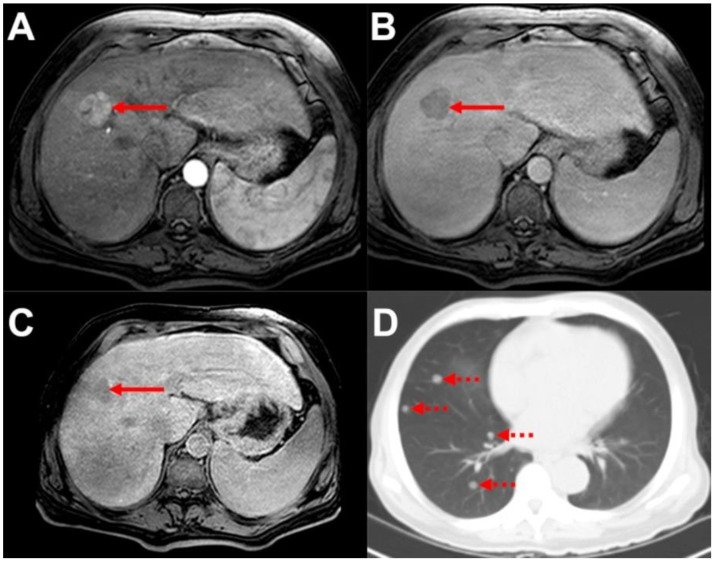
EHD leading to change in management in a patient with solitary HCC. (A): liver MRI, arterial phase and axial plane. (B): liver MRI, venous/transitional phase and axial plane. (C): liver MRI, hepatobiliary phase, and axial plane. (D): chest CT, lung window and axial plane. Note the solitary, typical HCC in liver segment VIII ((A–C), solid arrows) demonstrating arterial hypervascularization (A), venous/transitional phase washout (B), and hypointensity in the hepatobiliary phase ((C)—note is also made of visually reduced parenchymal contrast uptake due to impaired liver function). Based on hepatic disease, the treatment recommendation of RG1 was curative locoregional therapy. However, contrast-enhanced CT (D) revealed four pulmonary metastases in the right lung ((D), dashed arrows). The treatment recommendation was consequently changed to palliative systemic therapy.
